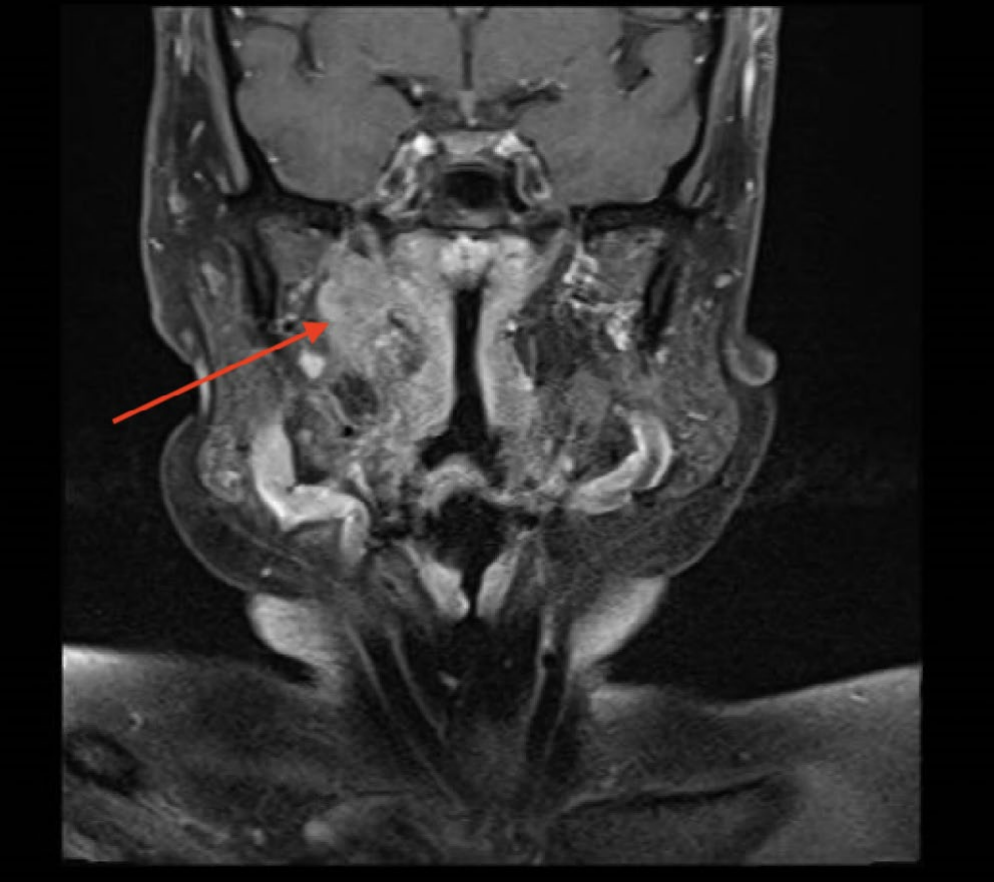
Preoperative T2‐weighted coronal MRI indicating an enhancing mass of the right parapharyngeal space (red arrow) with anteromedial displacement of the hypoglossal nerve and posterolateral displacement of the internal carotid artery.
